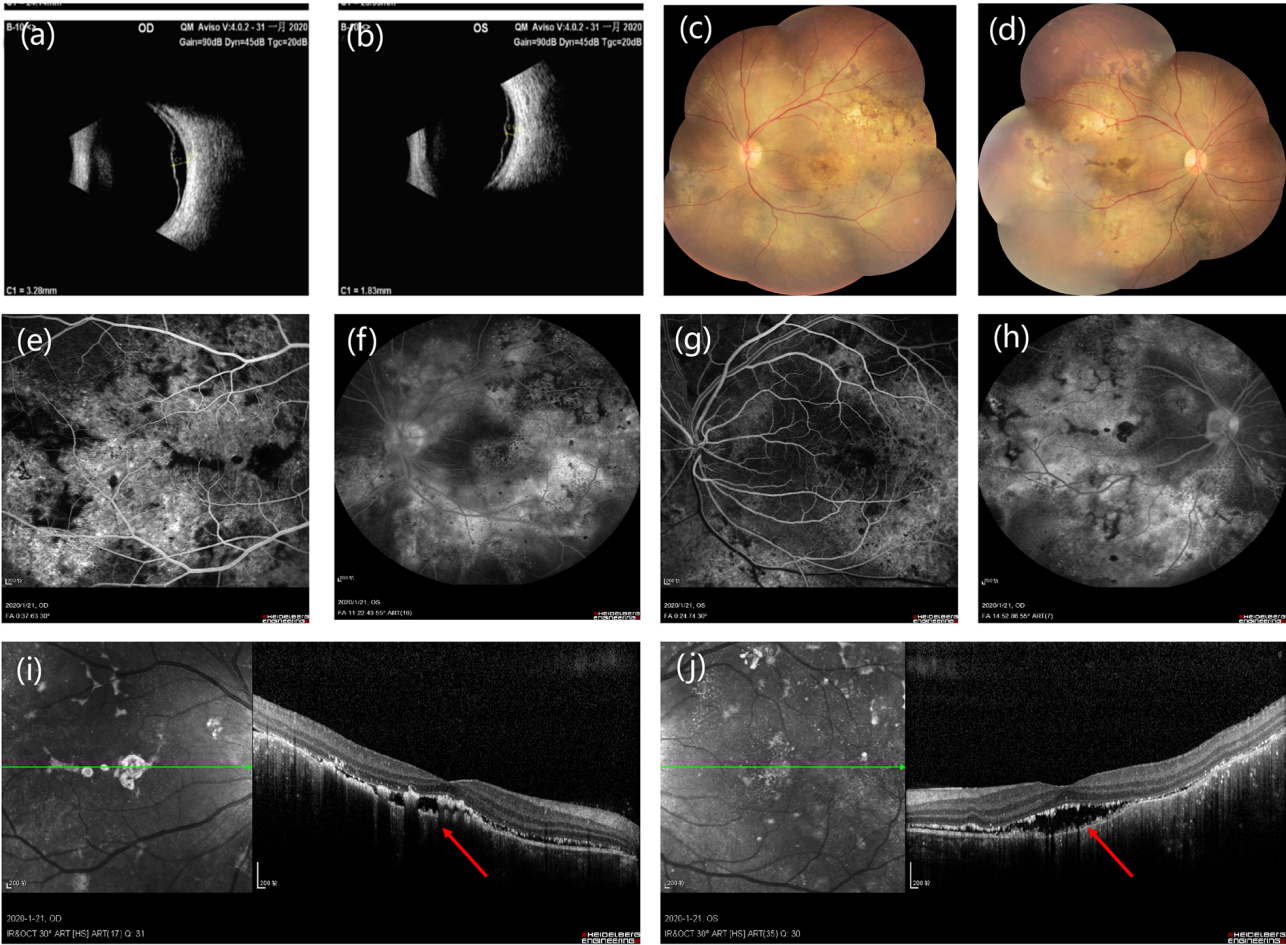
(A, B) Bilateral ultrasound images showing posterior retinal detachment in both eyes. (C, D) Fundus photographs showing large areas of depigmentation in retina. (E–H) Fluorescein fundus angiography (FFA) reveals early hypofluorescence of left (E) and right (G) side and late hyperfluorescence of left (F) and right (H) side in the optic disc area. (I, J) Optical coherence tomography of the left (I) and right (J) eye shows pockets of subretinal fluid.
